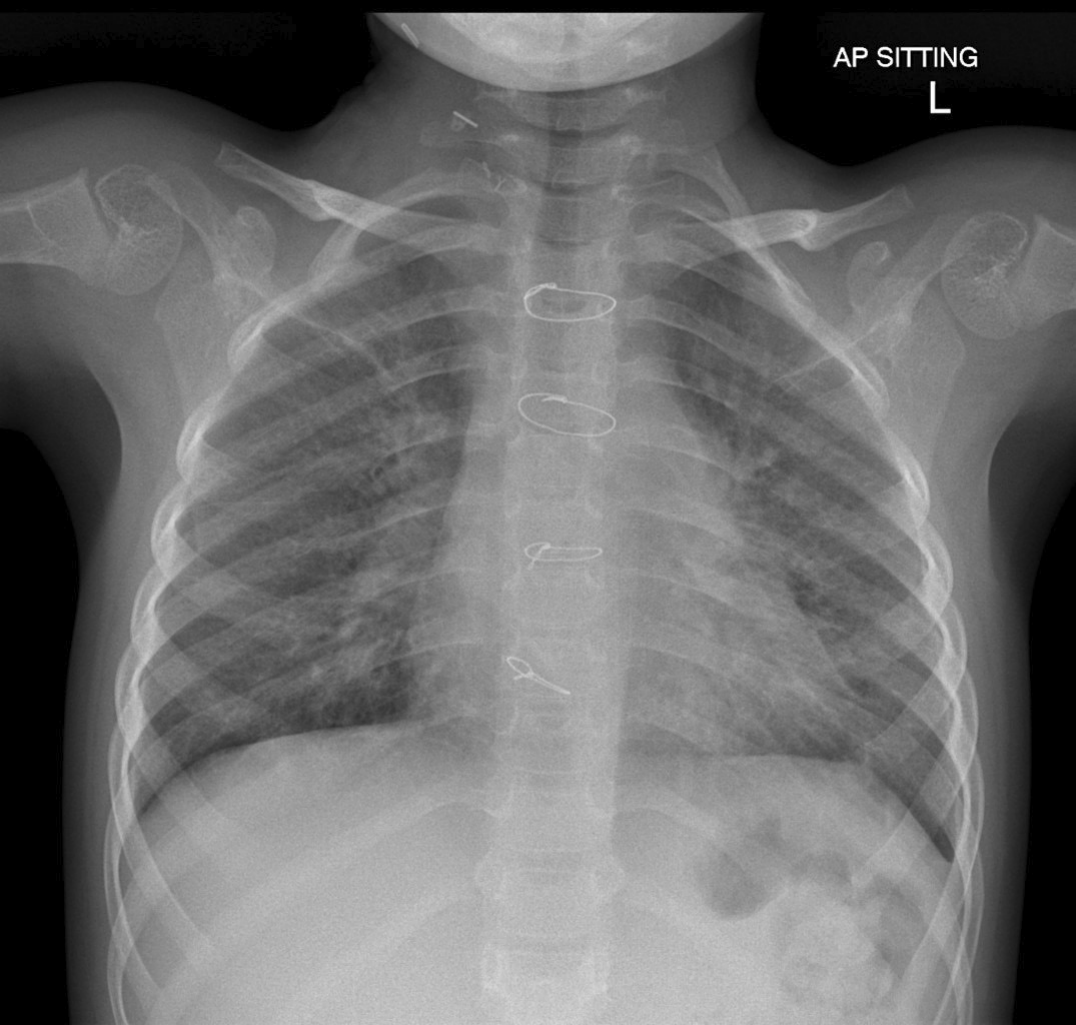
Chest radiograph performed post‐decannulation of tracheostomy 3 months post‐discharge
